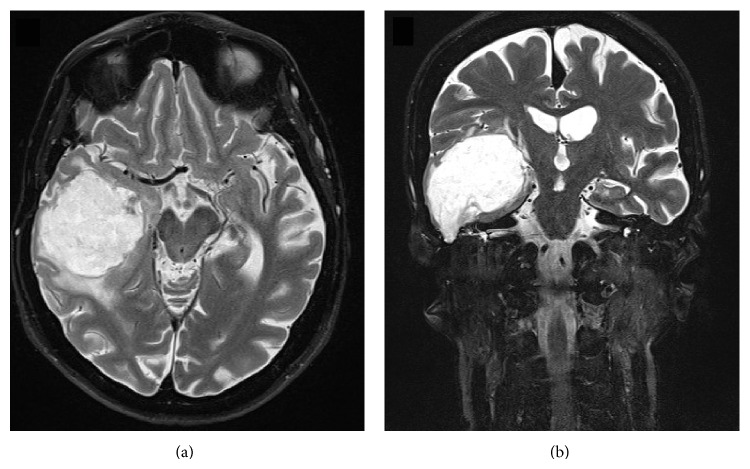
(a) T2-weighted MRI axial image demonstrates a smooth bordered heterogenous hyperintense mass approximately 55 mm × 48 mm with surrounding cytotoxic oedema of brain tissue. (b) T2-weighted MRI coronal image demonstrates the mass extending approximately 52 mm superiorly into the temporal lobe from the right middle ear via tegmen mastoideum defect.
